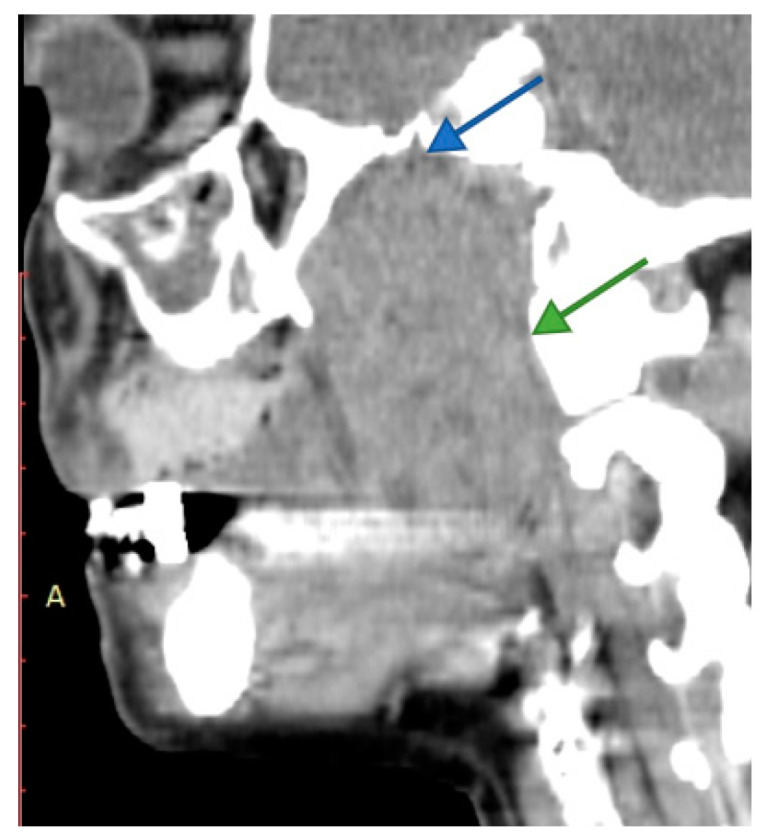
Cervical CT view in the sagittal plane showing an extensive facial nerve schwannoma in the right infratemporal fossa, retro maxillary, with the upper pole in contact with the base of the skull (blue arrow) and the posterior pole in the prevertebral plane (green arrow).
